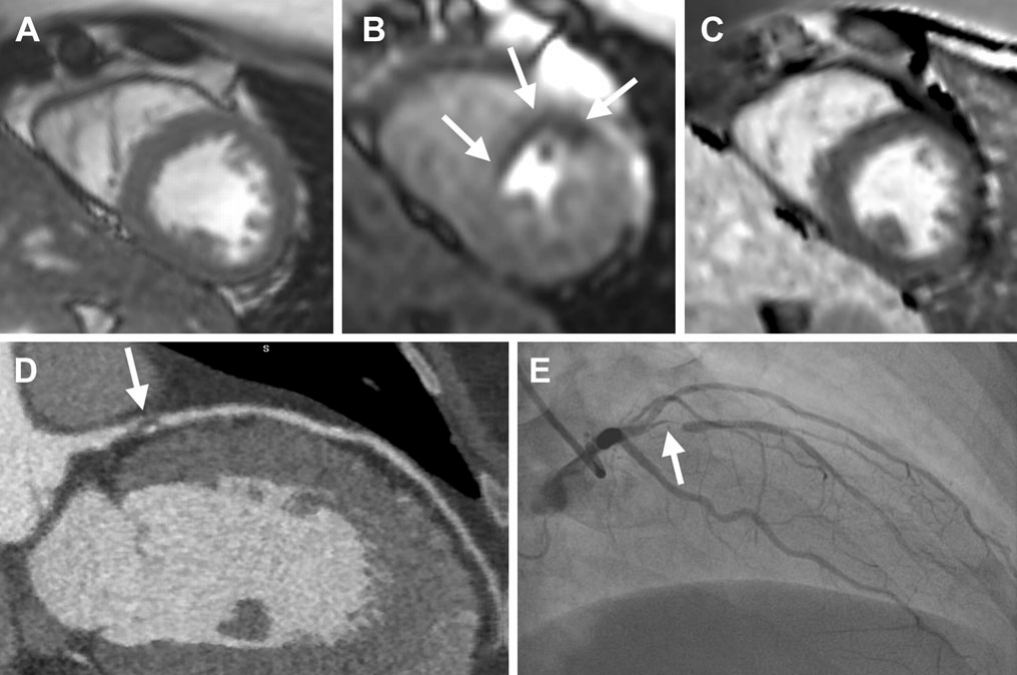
Example of concordant positive findings on CMR and CTA from a 46 year old female with no prior cardiovascular history presenting with intermediate pre-test probability for CAD and an equivocal nuclear SPECT study. The short axis cine CMR showed normal wall thickness (a), an anterior and anteroseptal stress induced perfusion defect (b, *arrows*) without evidence of myocardial infarction on late gadolinium enhancement imaging (c). The CTA showed an obstructive mixed calcified and non-calcified stenosis of the proximal left anterior descending (LAD) coronary artery (d, *arrow*). Invasive angiography confirmed a severe proximal stenosis of the LAD (e, *arrow*)
